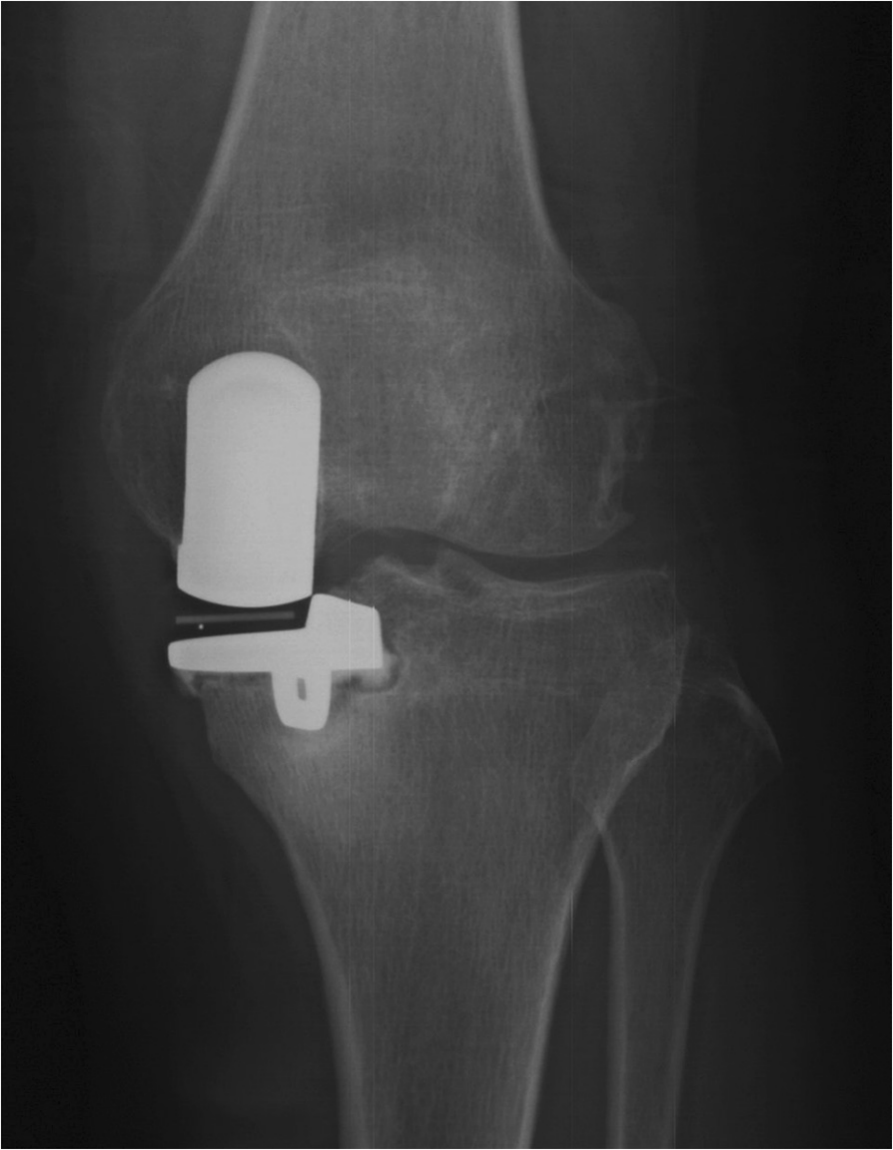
Anteroposterior X-ray of postoperative UKA infection.
